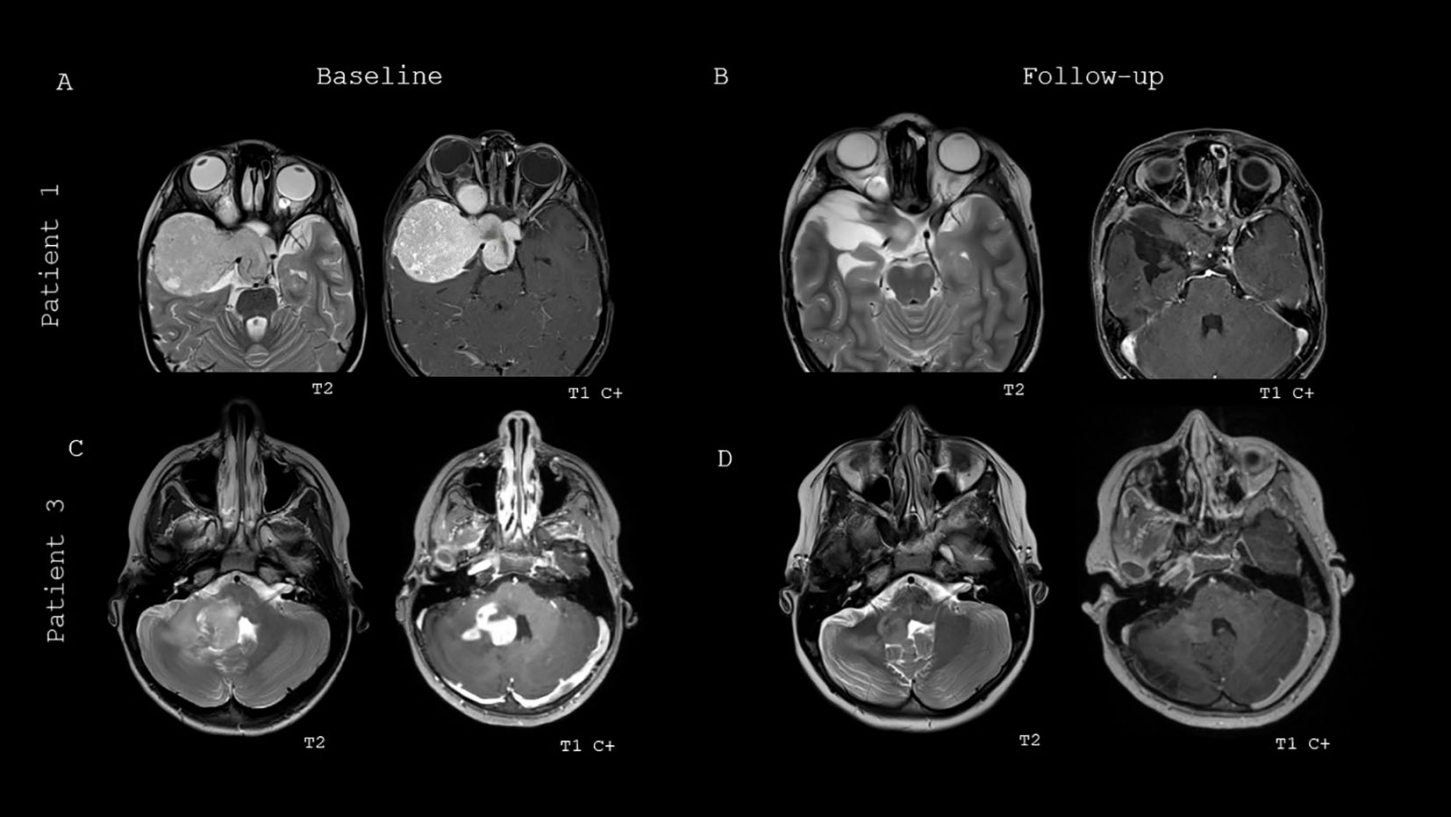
Baseline and best response MRI brain imaging of two patients treated with BRAFi monotherapy. (A) Baseline MRI brain imaging of patient 1 prior to start of BRAFi therapy. (B) Best response imaging of patient 1 on BRAFi therapy. (C) Baseline MRI brain imaging of patient 3 prior to start of BRAFi therapy. (D) Best response imaging of patient 3 on BRAFi therapy.
